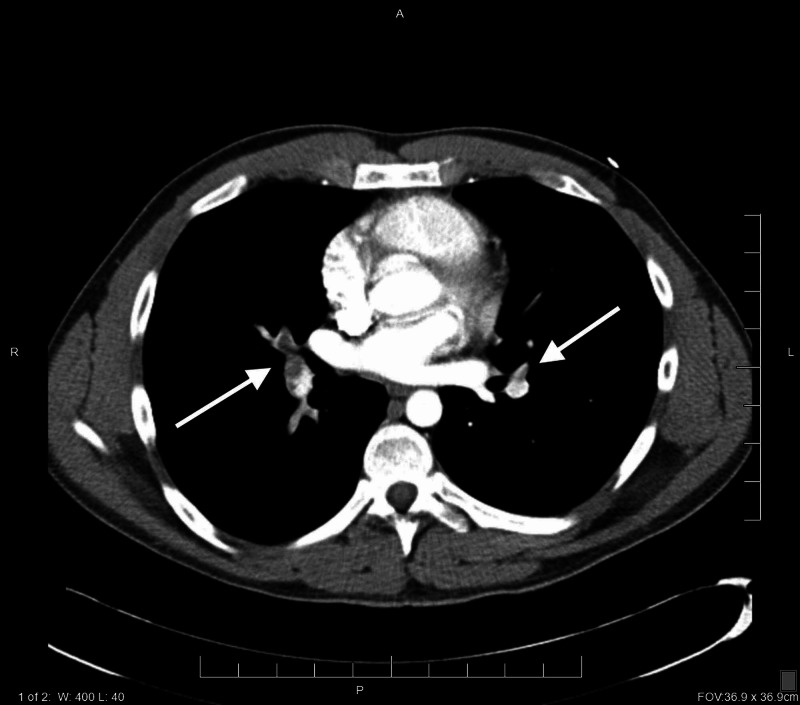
CT angiogram demonstrating filling defects in branches of pulmonary arteries bilaterally in keeping with pulmonary emboli.
